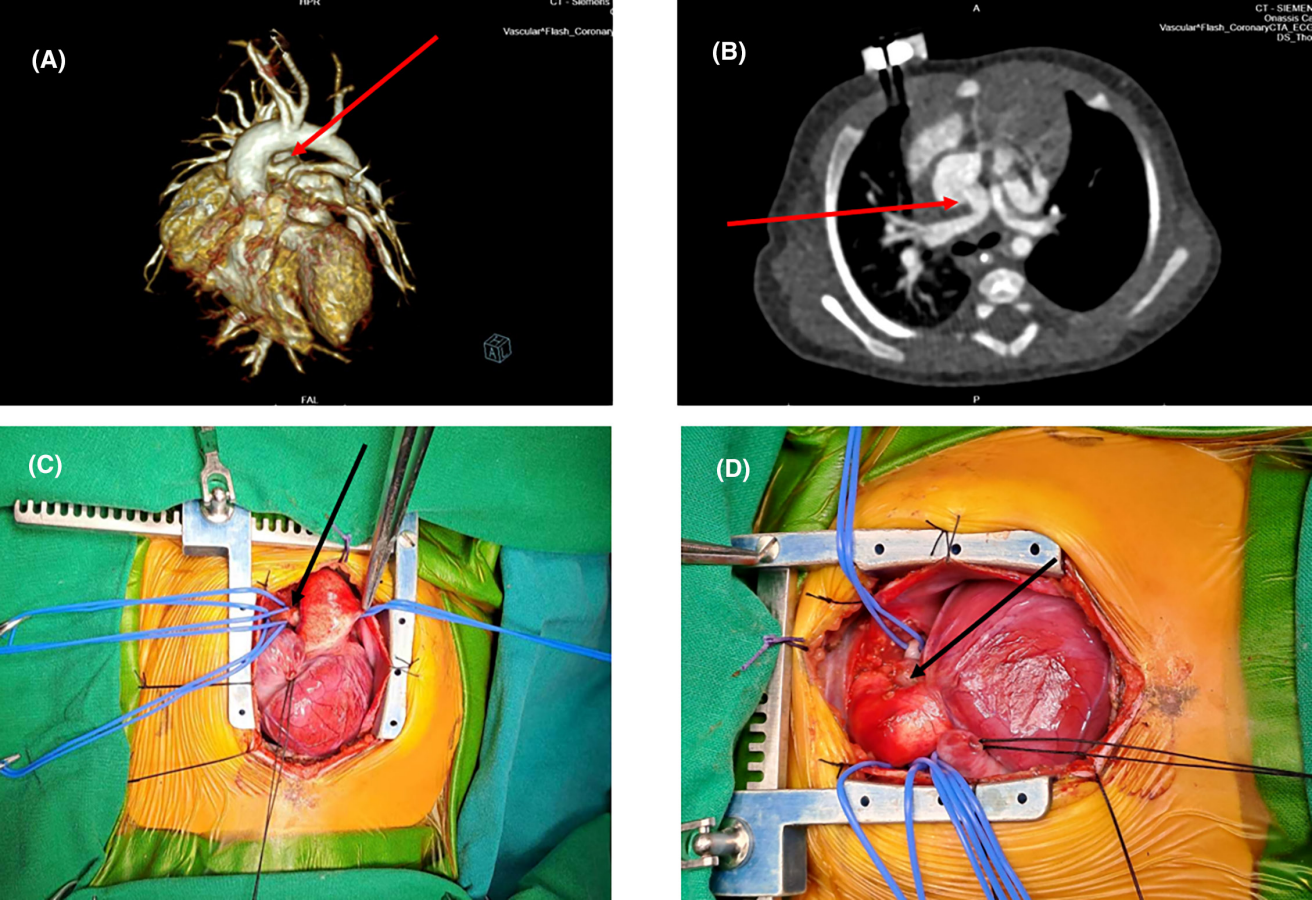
Computed tomography scan with 3D reconstruction and intraoperative images; showing the anomalous right pulmonary artery originating from the ascending aorta (A, B red arrow and C, black arrow). Inlet image (D) showing the atretic pulmonary artery (black arrow).
